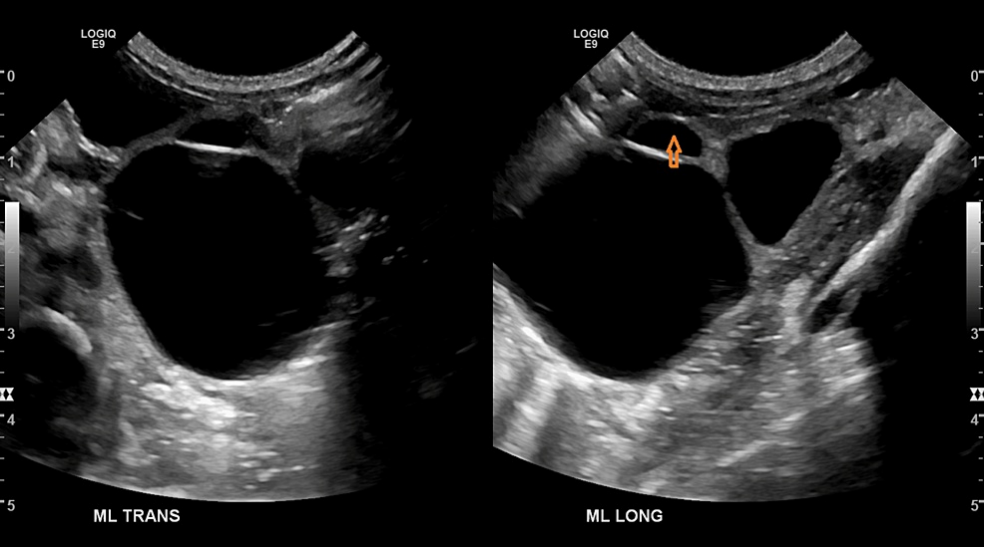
The arrow in the picture points to the multicystic dysplastic kidney that was identified in the ultrasound.
